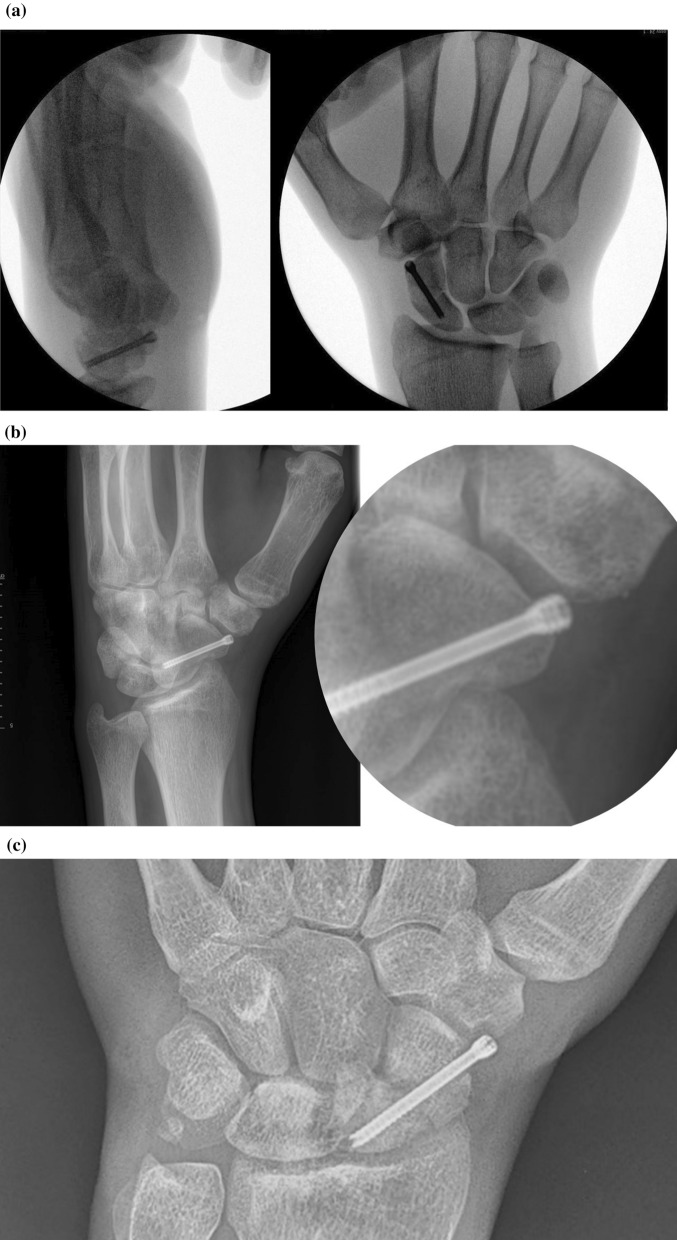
A Standard anteroposterior and lateral fluoroscopy views of a percutaneous cannulated screw fixation of a scaphoid waist fracture showing minimal STT screw penetration. B Postoperative semi-pronated radiograph showing significant distal articular screw penetration and absence of screw thread purchase in the distal fragment. C Another example of significant distal articular screw penetration with subsequent delayed union of a scaphoid waist fracture
